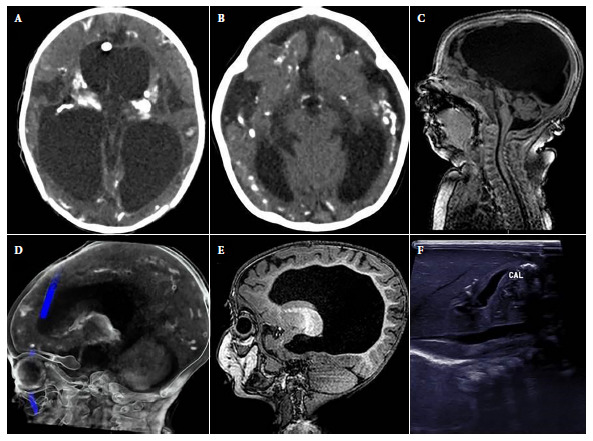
Abnormal findings in images of the cases. A). hydrocephalus and intracranial calcifications in cross-sectional brain computerized tomography. B). Decreased brain parenchymal volume with ventricular dilatation, microcephaly and intracranial calcifications in cross section of brain computerized tomography. C). Cerebellar hypoplasia in sagittal section of brain MRI. D). Intracranial calcifications in sagittal section of cerebral computerized tomography. E). Dysgenesis of the corpus callosum in sagittal section of brain MRI. F). Hepatic calcifications in abdominal ultrasound.
